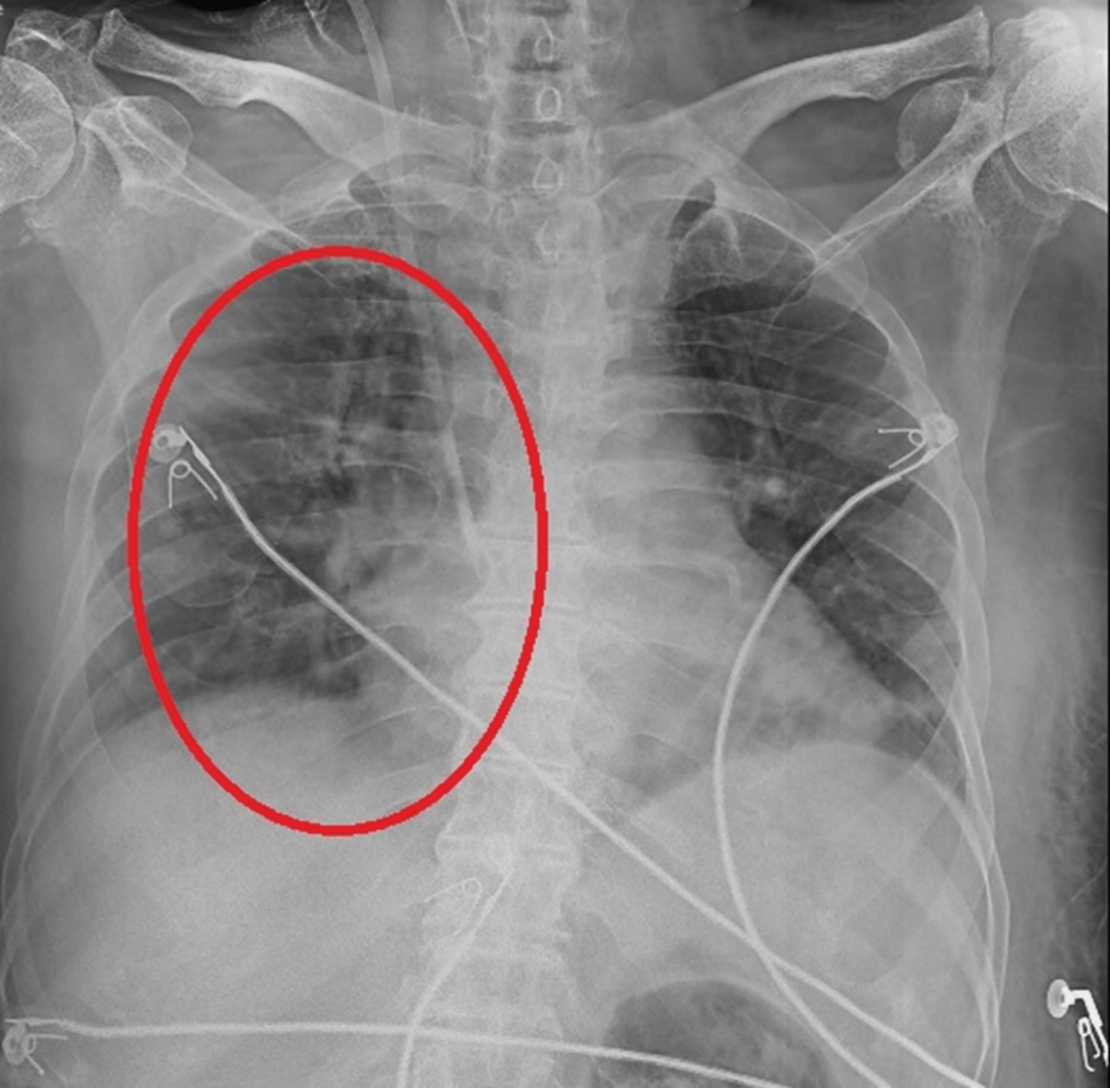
Pre-physiotherapy rehabilitation X-ray.
